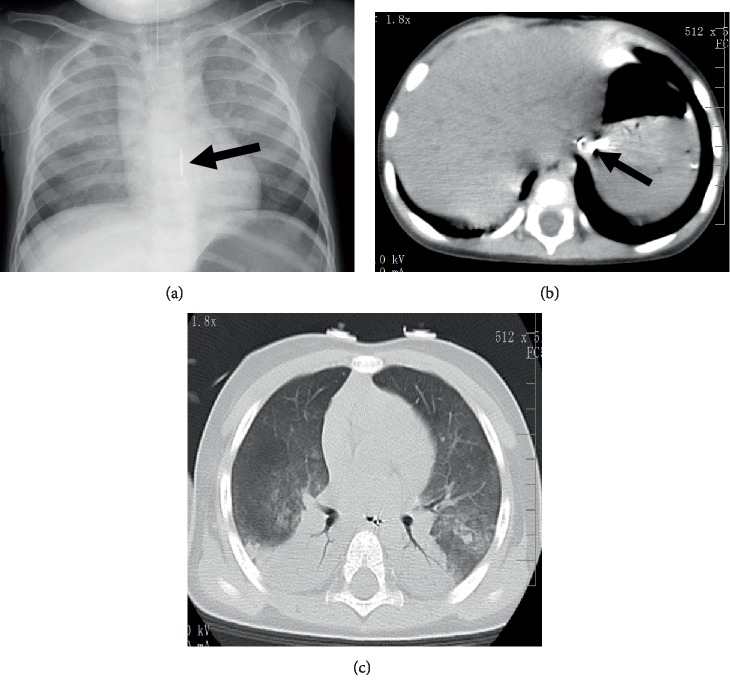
Imaging findings at hospital admission. (a) Chest X-ray; (b, c) chest computed tomography.
